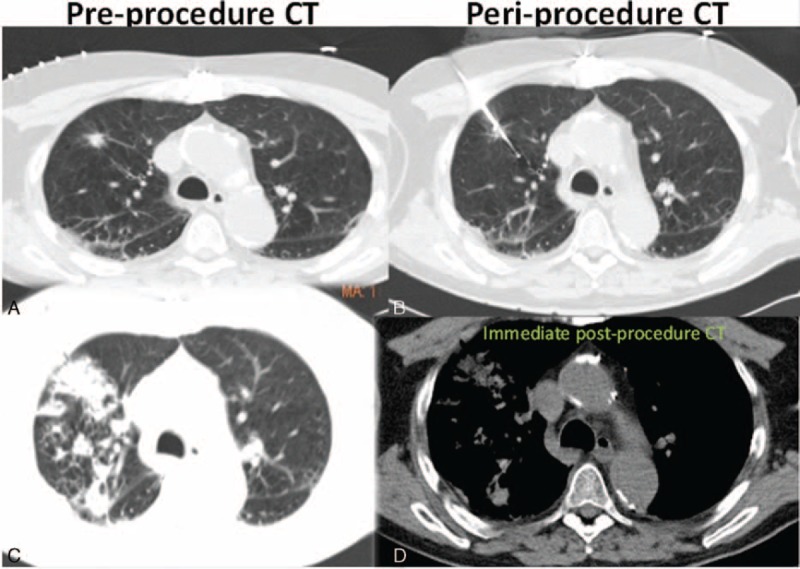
Shows a typical patient status postcryoablation procedure. The precontrast axial CT. A shows a biopsy proven adenocarcinoma in the right upper lobe. B shows the percutaneous cryoablation device centered in the lung cancer. C and D both show the postprocedural hemorrhage which is typically seen in cryotherapy. Please note that a freeze-thaw-freeze cycle was performed on all these patients for continuity of care and not a “triple freeze” protocol. CT = computed tomography.
